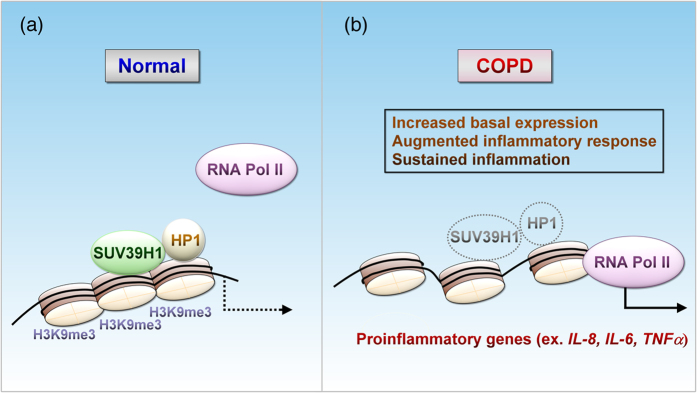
A scheme of SUV39H1 down-regulation involved in the dysregulation of inflammation in COPD. Reduction of SUV39H1 modulation releases repressive chromatins and enhances cytokine secretion to the inflammatory environment in COPD, which potentially amplifies the inflammation response and disease progression.(a) In normal subjects, SUV39H1 mediates the repressive chromatin state of pro-inflammation genes, e.g., IL-8, IL-6, and TNFα, but not the one encoding Th2 cytokines, through an interplay with H3K9me3 and HP1. By keeping these genes in check, cells are stable and the inflammatory response is tightly controlled.(b) In patients with COPD, a profound reduction in SUV39H1 releases the repression of such genes and resets the production of cytokines to a higher level in the chronic inflammatory milieu. Upon further stimulation(ex. oxidative stress), transactivation of these genes results in prolonged enhancement in an unchecked manner.
